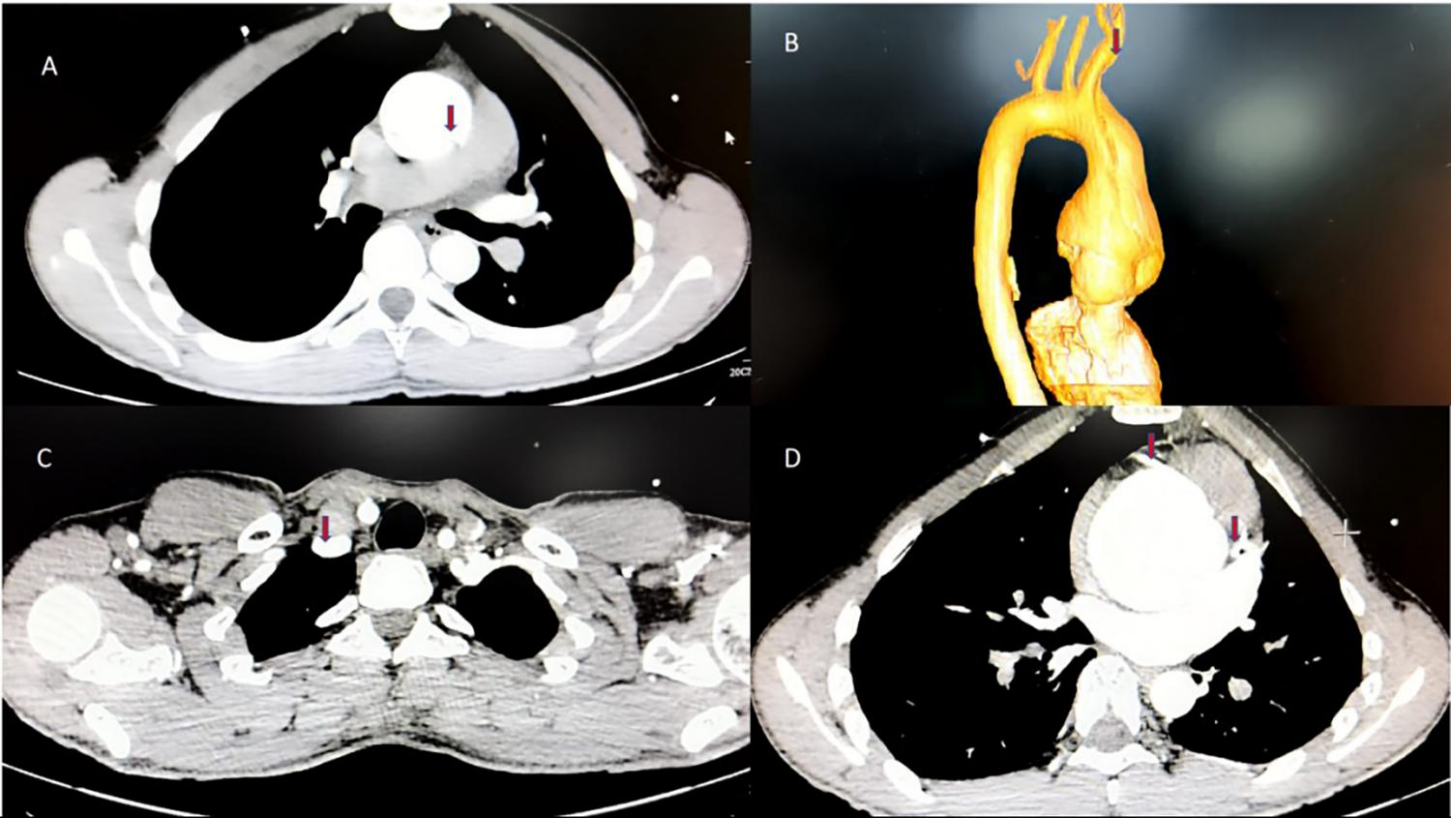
CTA of the thoracoabdominal aorta performed at the local hospital. (A) The patient presented with a “pigeon chest” deformity, and a significant aneurysmal dilation of the aortic root accompanied by AD, with a diameter of approximately 62 mm at its widest point; (B) the three-dimensional imaging showed an AD involving the proximal portion of the brachiocephalic artery; (C) the right subclavian artery is well-filled, and the dissection does not extend to it; (D) both left and right coronary arteries are well-filled, with no hematoma compression, ruling out the presence of acute myocardial infarction at this time.
